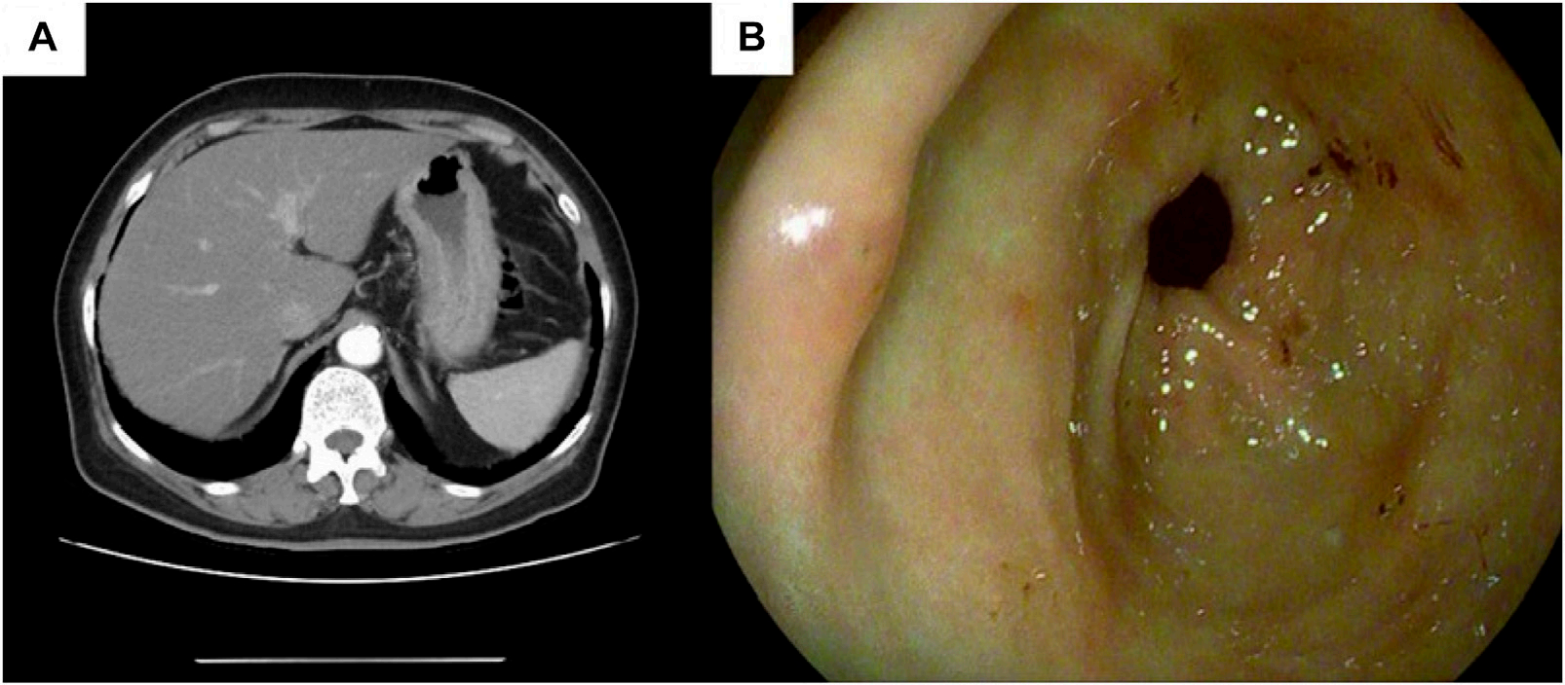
Gastric endoscopy and CT images of the patient. (A) Abdominal CT shows gastric wall thickening,the thickest part of which was about 2.3 cm in diameter, involving the whole circumference of the lumen, with a length of about 5 cm; (B) Endoscopy prompts chronic superficial atrophic gastritis.
